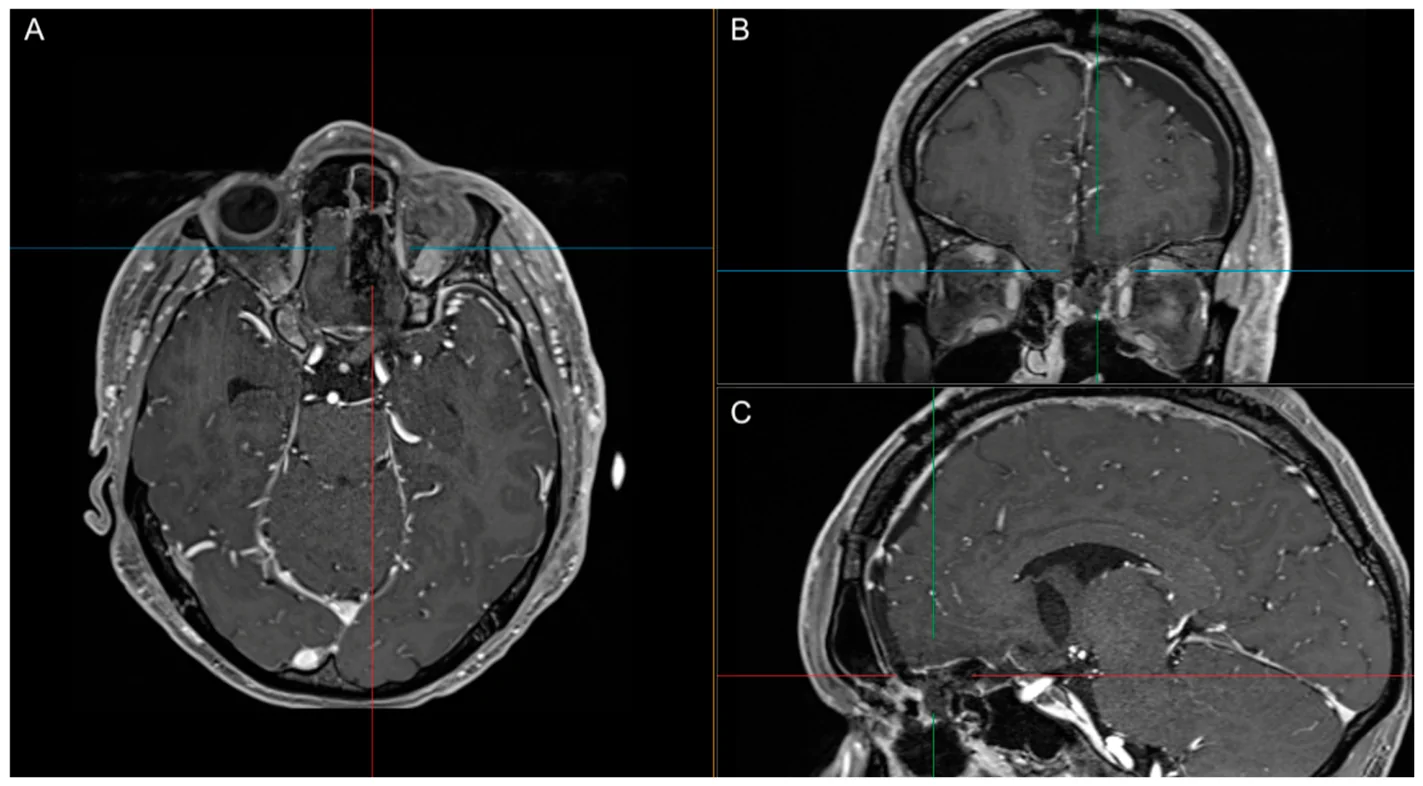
Postoperative T1 post-contrast MRI, showing complete resection of the lesion. (A) Axial T1 post-contrast image. (B) Coronal T1 post-contrast image. (C) Sagittal T1 post-contrast image.
